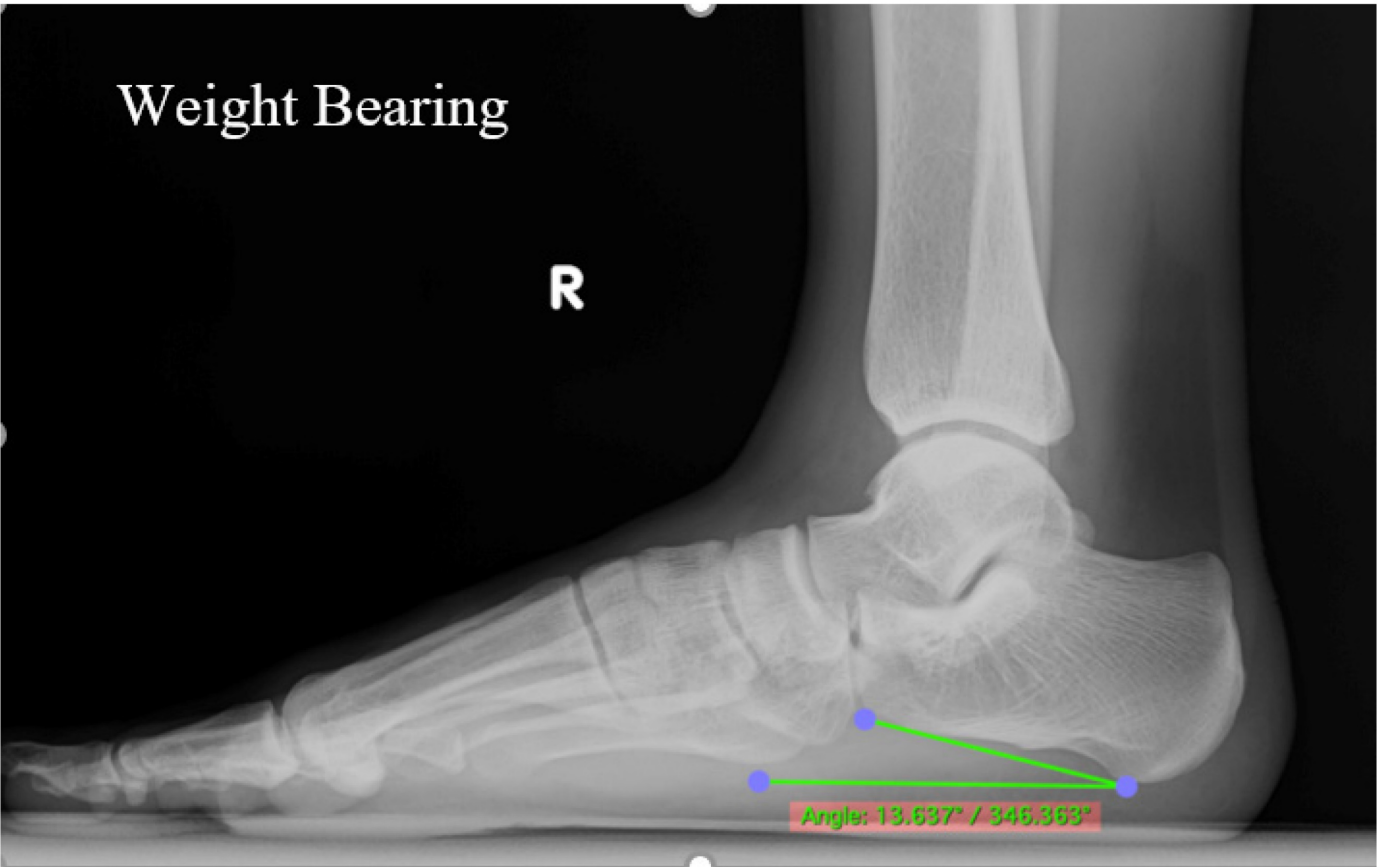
Lateral radiograph shows the calcaneal inclination angle.
